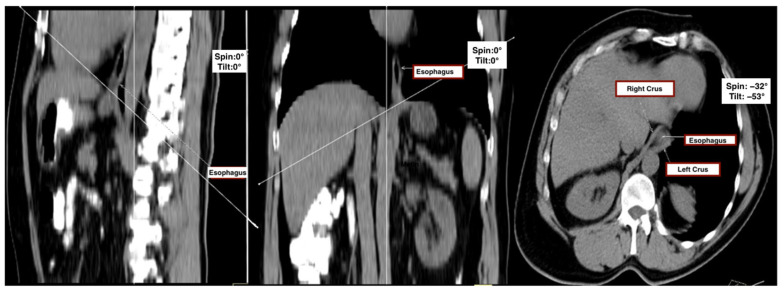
Multiplanar CT evaluation of the esophageal hiatus. Standardized sagittal (left), coronal (middle), and oblique axial (right) MDCT reconstructions used for anatomical localization and morphometric analysis of the esophageal hiatus. The esophagus and diaphragmatic crura (right and left) are labeled. The oblique axial plane was obtained by aligning with the diaphragmatic crura and orthogonal to the sagittal axis to best visualize the hiatal components for surface area measurement.
